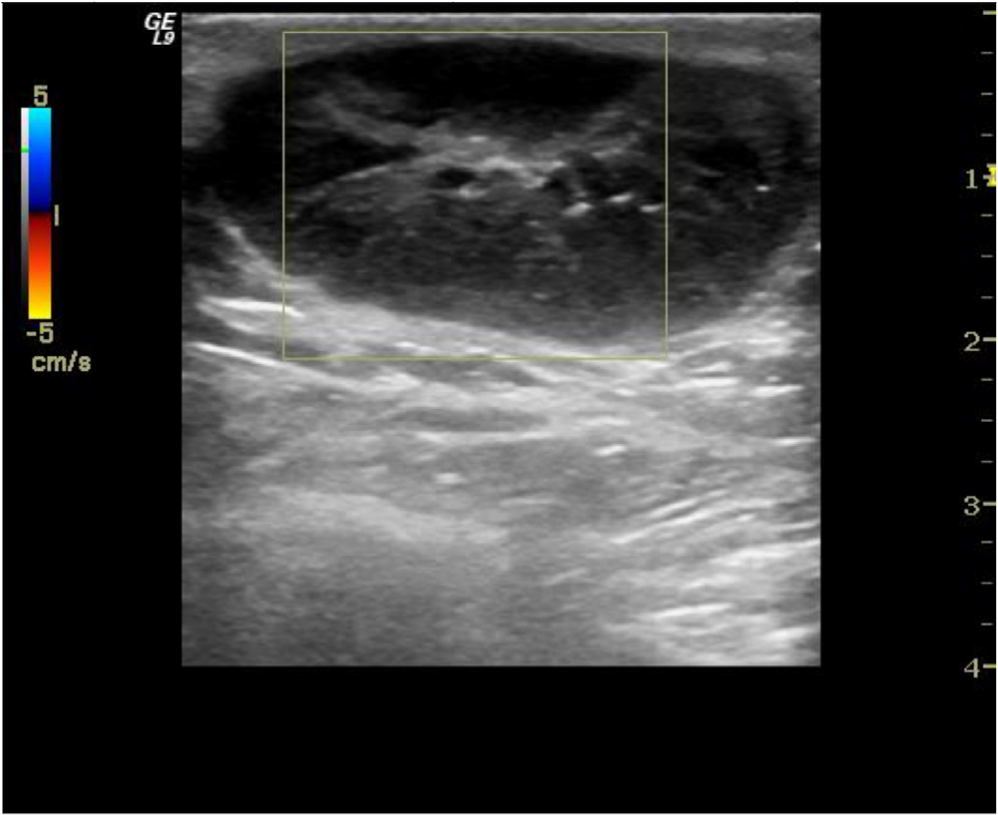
Axial sonogram shows well-circumscribed, hypoechoic lesion with posterior acoustic enhancement in the posterior of nipple. No vascular signal was noted in color box.
